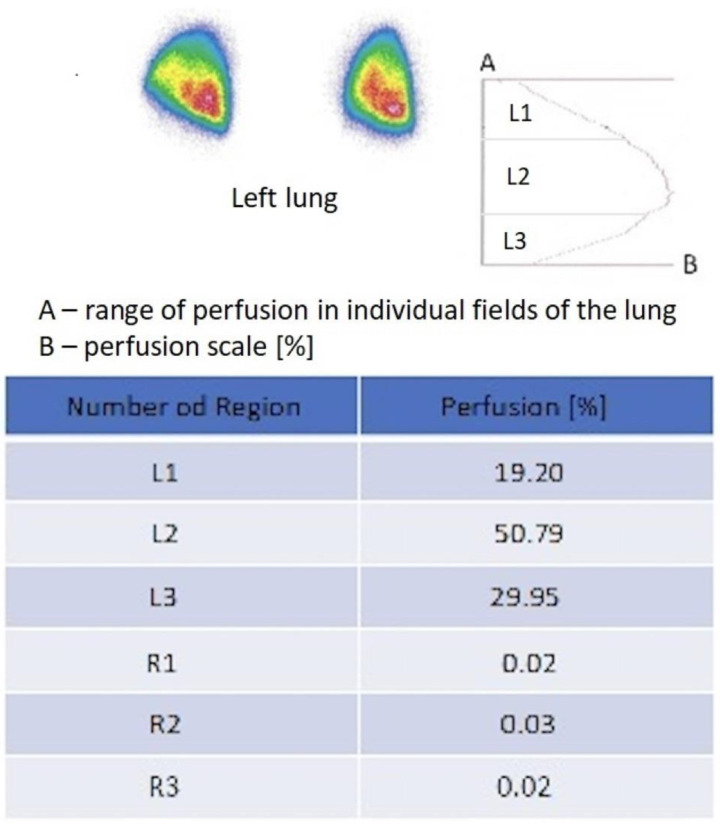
Original model scintigraphic report of left lung after right pneumonectomy. The perfusion map [%] shows scale of perfusion of 3 areas of the left lung: L1-upper, L2-middle, and L3-lower field and lack perfusion of the right lung (R1, R2, R3). The report is completed by isotope accumulation intensity curve plot. Red color indicates the area with the highest flow intensity, while blue indicates the area with the lowest flow. Yellow and green indicate areas corresponding to intermediate flow values.
